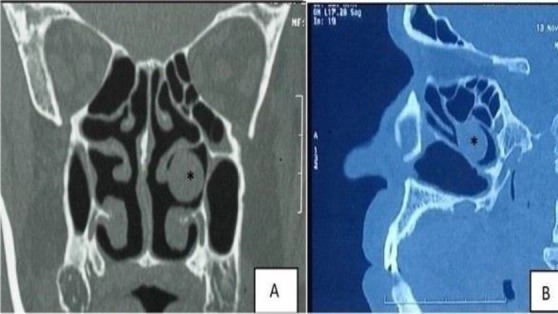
Computed tomography of osteomeatal complex; A) coronal section and B) sagittal section showing globular polypoidal mass attached to left middle turbinate lying in left middle meatus region
